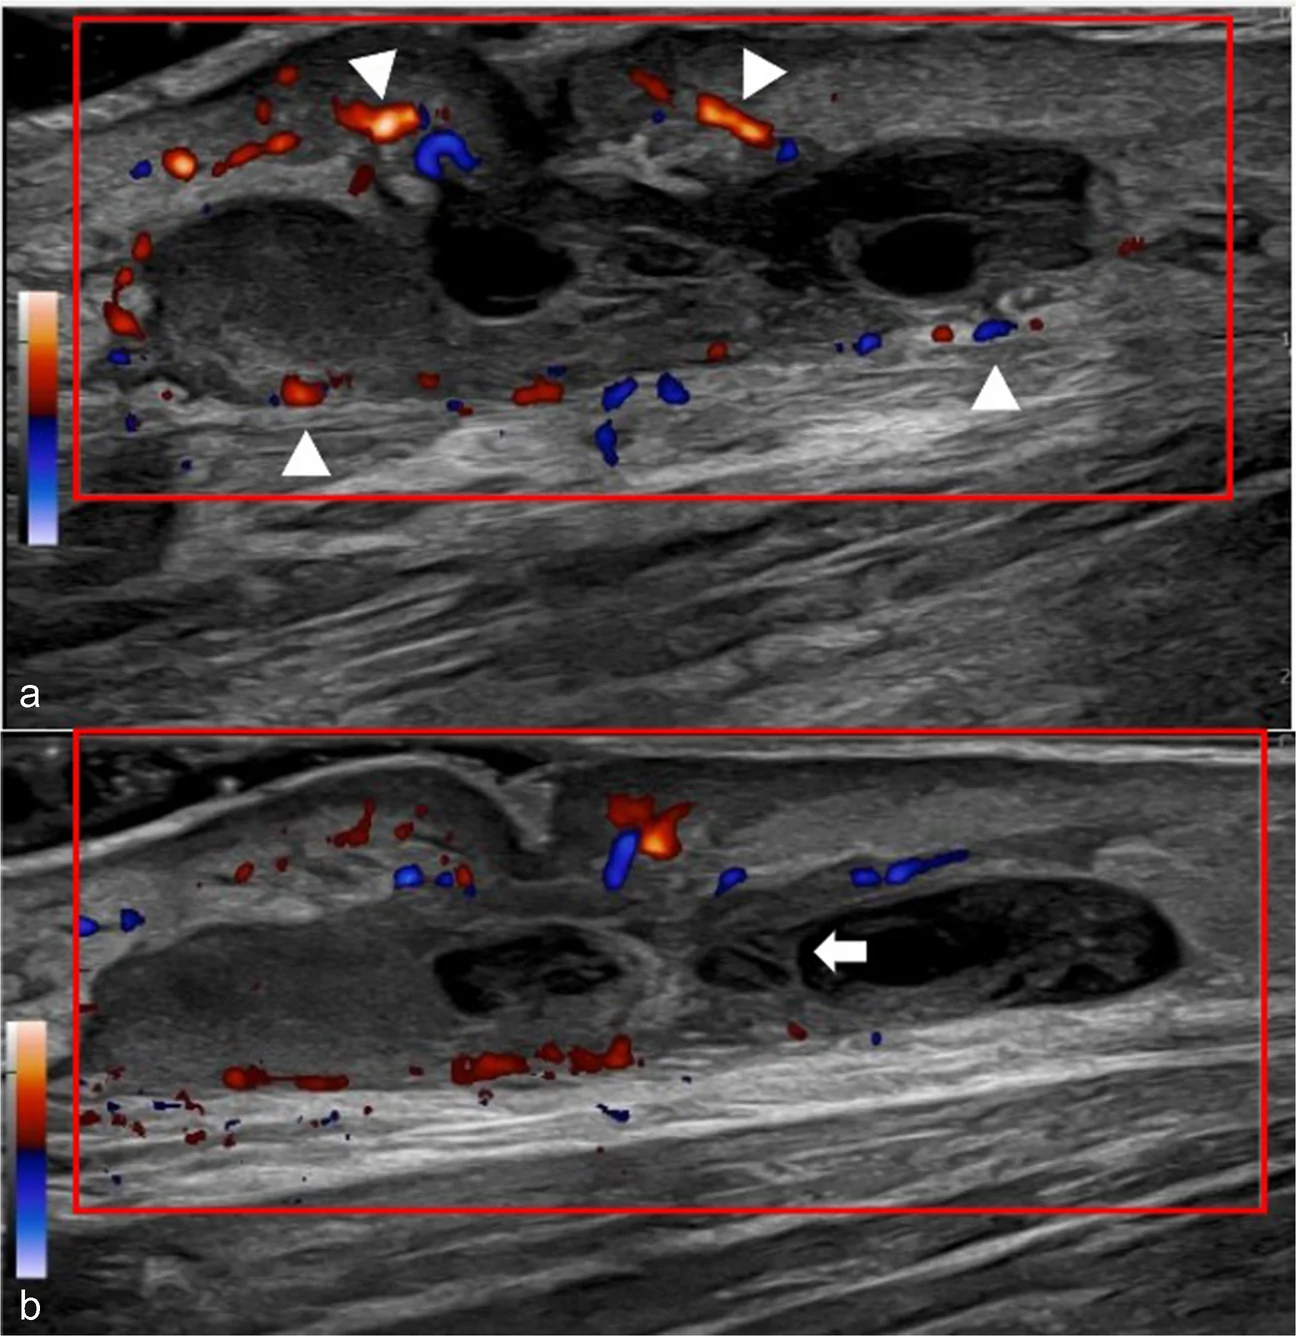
a, b Ultrasound images demonstrate a predominantly cystic subcutaneous lesion with thick internal septations (arrow) and peripheral vascularity on color Doppler imaging (arrowheads). The lesion is confined to the subcutaneous tissue, with preservation of the adjacent musculature
